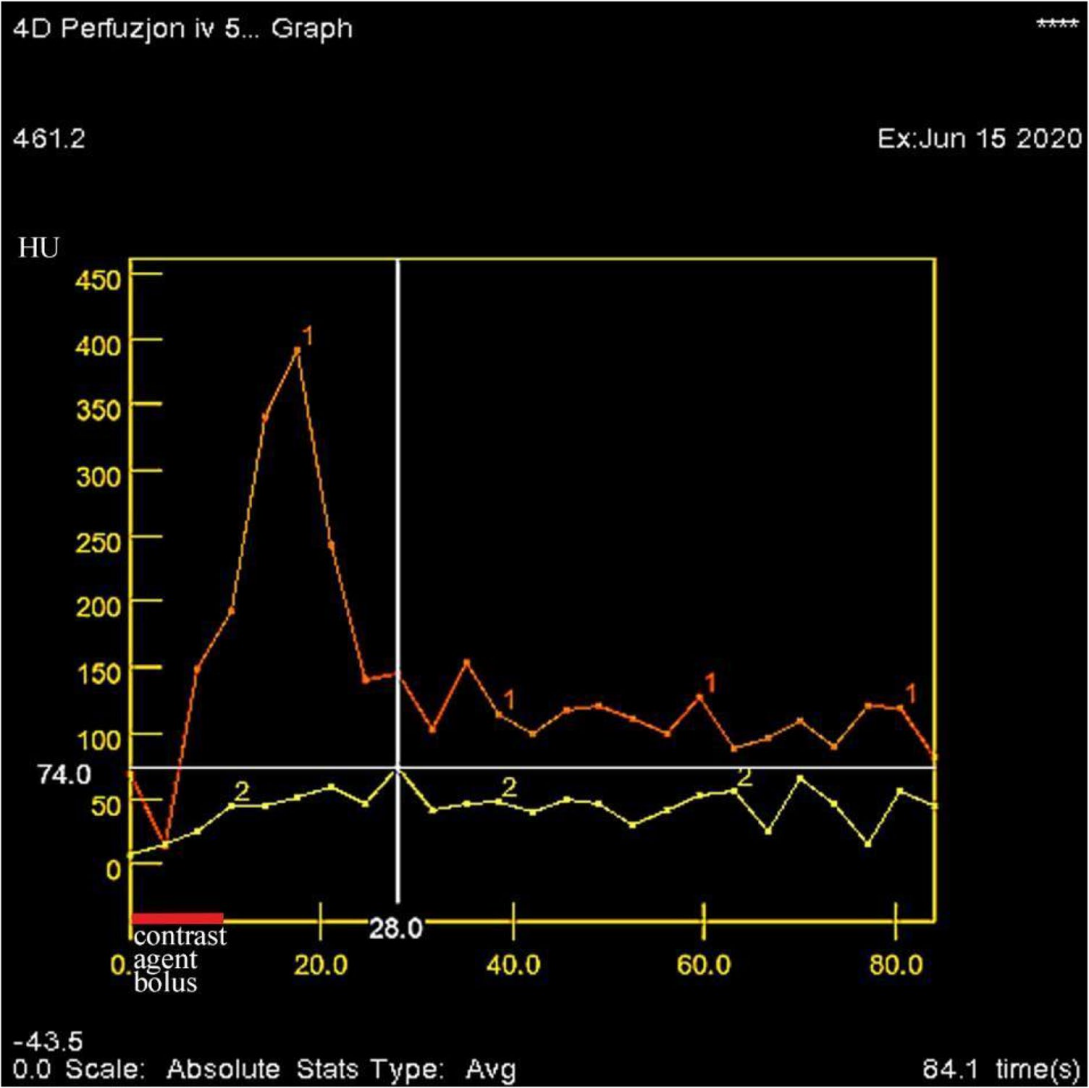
An example of CTP scanning*.* The contrast enhancement of the aorta is marked in red and the flow in the SPN is marked in yellow. The contrast bolus lasts for 10 s and begins simultaneously with the commencement of the scan. The greatest contrast enhancement of the tumor to + 74 HU is observed 28 s after contrast administration. *CTP* perfusion computed tomography.
